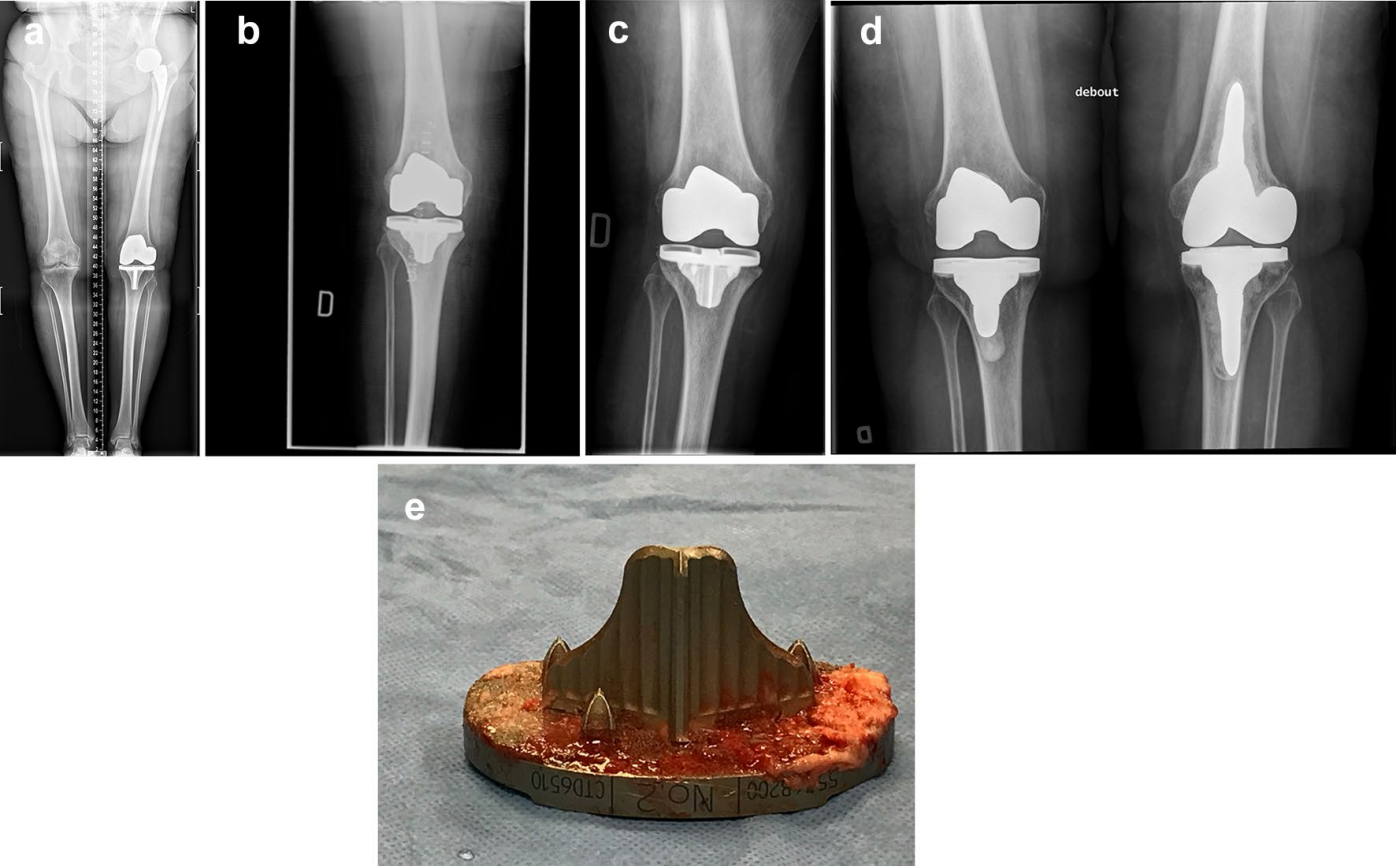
a Pre-op long leg AP view radiograph of a 76-year-old female with severe medial OA where LDFA is measured at 87.5° (valgus) and MPTA at 88.1° (varus), leading to an arithmetic HKA of 0.6°. b Immediate post-op AP radiograph showing uncemented TKA implants in acceptable orientation: 88.0° LDFA, 0° MPTA and arithmetic HKA of 2.0°. c Patient sustained a fall in stairs 4 weeks after surgery. Sudden and persisting pain and swelling were present. This is an AP view radiograph, 8 weeks post-op, showing a 5° valgus shift of the tibial implant (MPTA changed from 90° to 95° (LDFA was maintained at 88.0°). d Patient being unsatisfied with her lower limb alignment (HKA: 7° valgus) and having medial knee pain (medial collateral ligament over tensioned), she requested a TKA revision surgery. During revision procedure, the femoral implant was well fixed and considered well aligned. Tibial implant was revised alone, changing its orientation and using a cemented version. AP view radiograph post revision showing tibial implant’s MPTA at 88.0°, combined leading to an arithmetic HKA of 0° when combined with the femoral implant LDFA of 88.0°. e Removal of the well-fixed uncemented tibial implant was demanding, especially to break the osseous bonding behind the keel. Here is a photograph of the removed uncemented tibial implant where cancellous bone attachment is observed on the whole porous surface
